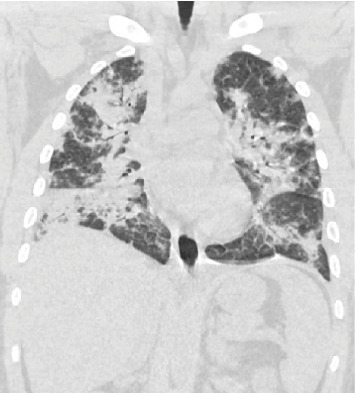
CT of the chest without contrast demonstrating patchy bilateral ground-glass opacities and small bilateral pleural effusions.
